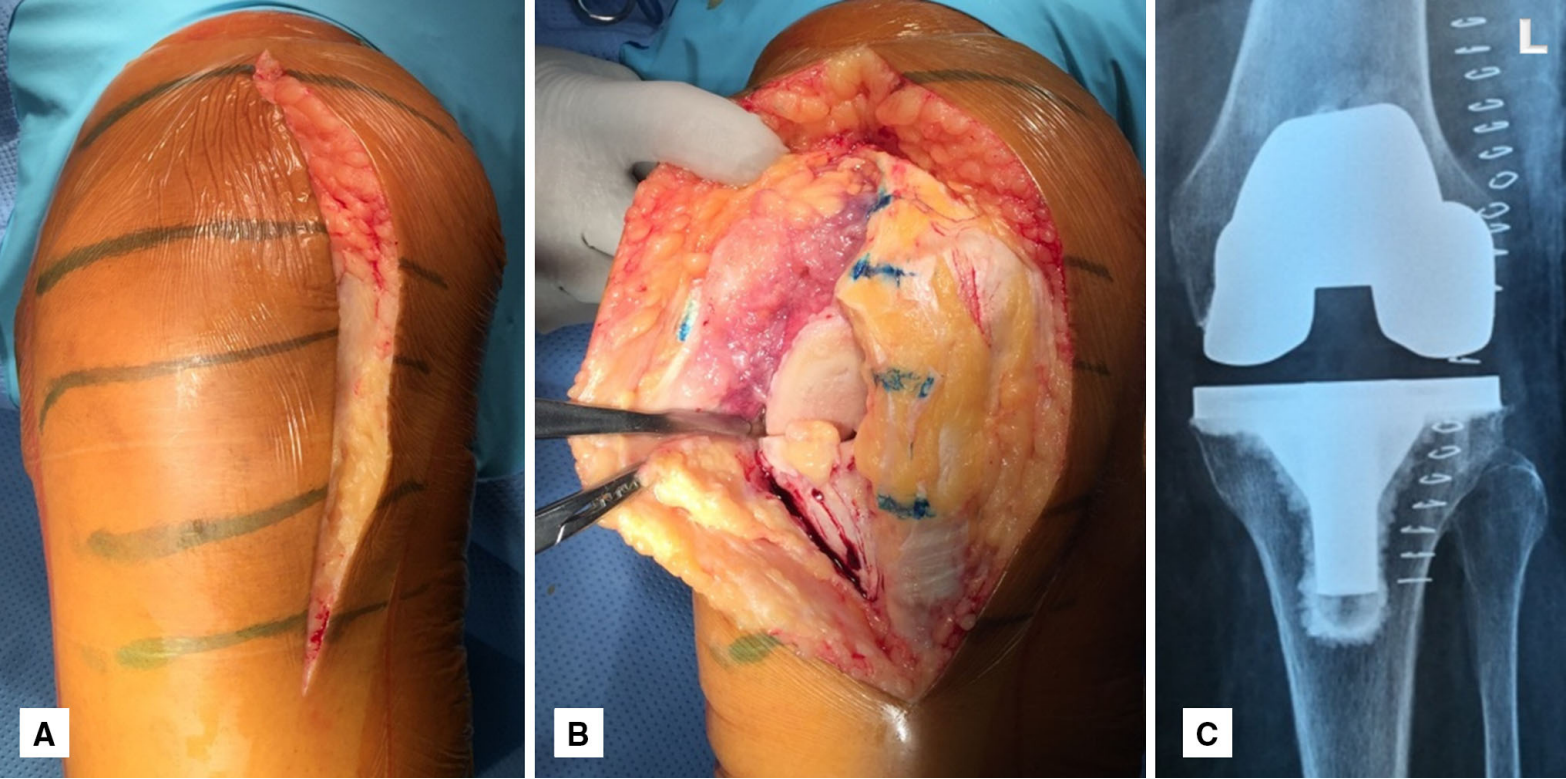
Photograph of a left knee showing a anterolateral skin incision, b medial arthrotomy and the raised medial flap, and c postoperative radiograph of the same patient
